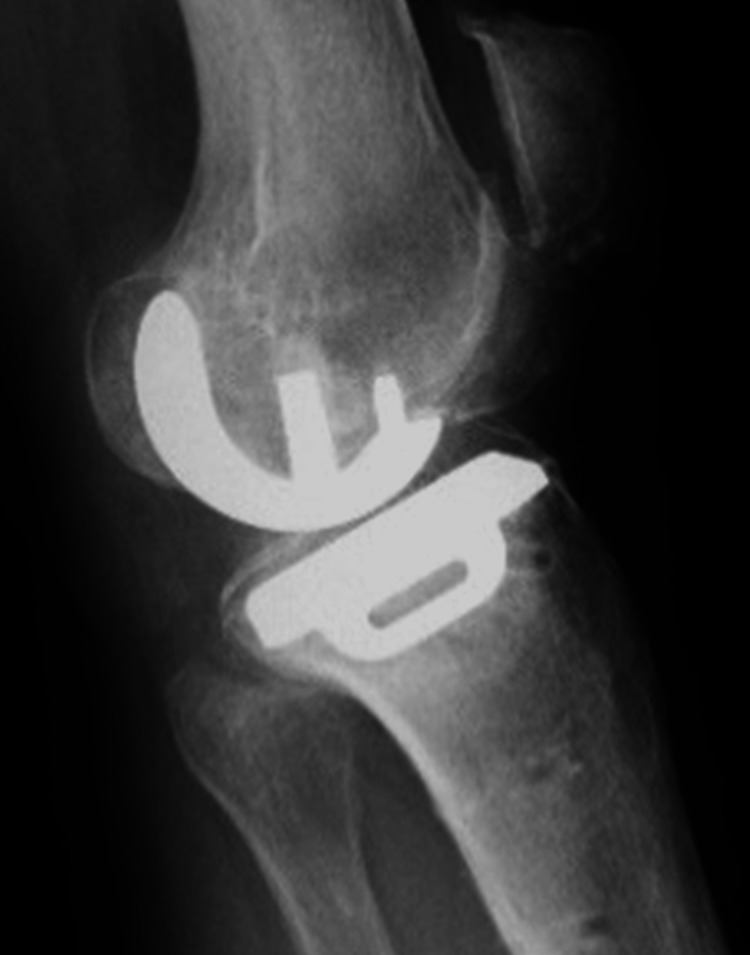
Postoperative radiography after plate removal (lateral view)
